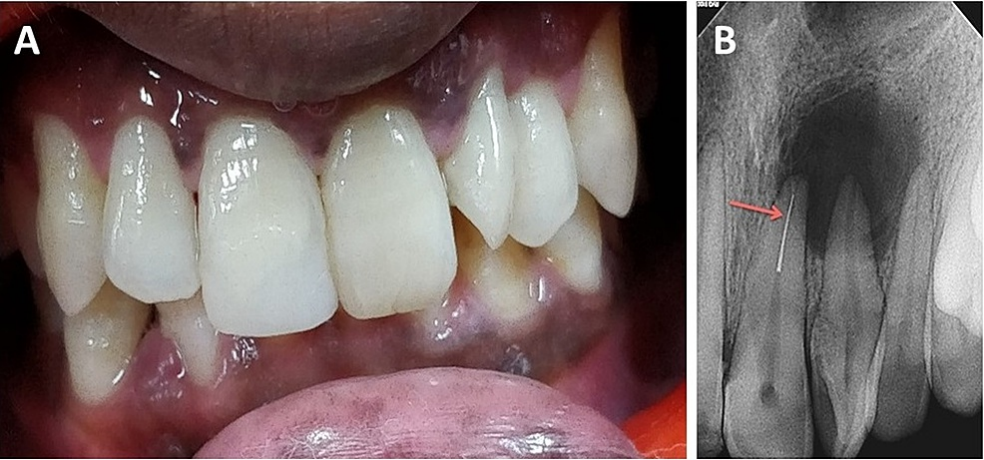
Intraoral photograph and radiograph A: Intraoral photograph showing slightly discolored left central incisor. A well-formed, rotated supernumerary tooth can be seen between the left central and lateral incisor. B: Radiograph showing the separated instrument in the apical third of central incisor (depicted by arrow). Large periapical lesions can be seen associated with central incisor and supernumerary tooth.
